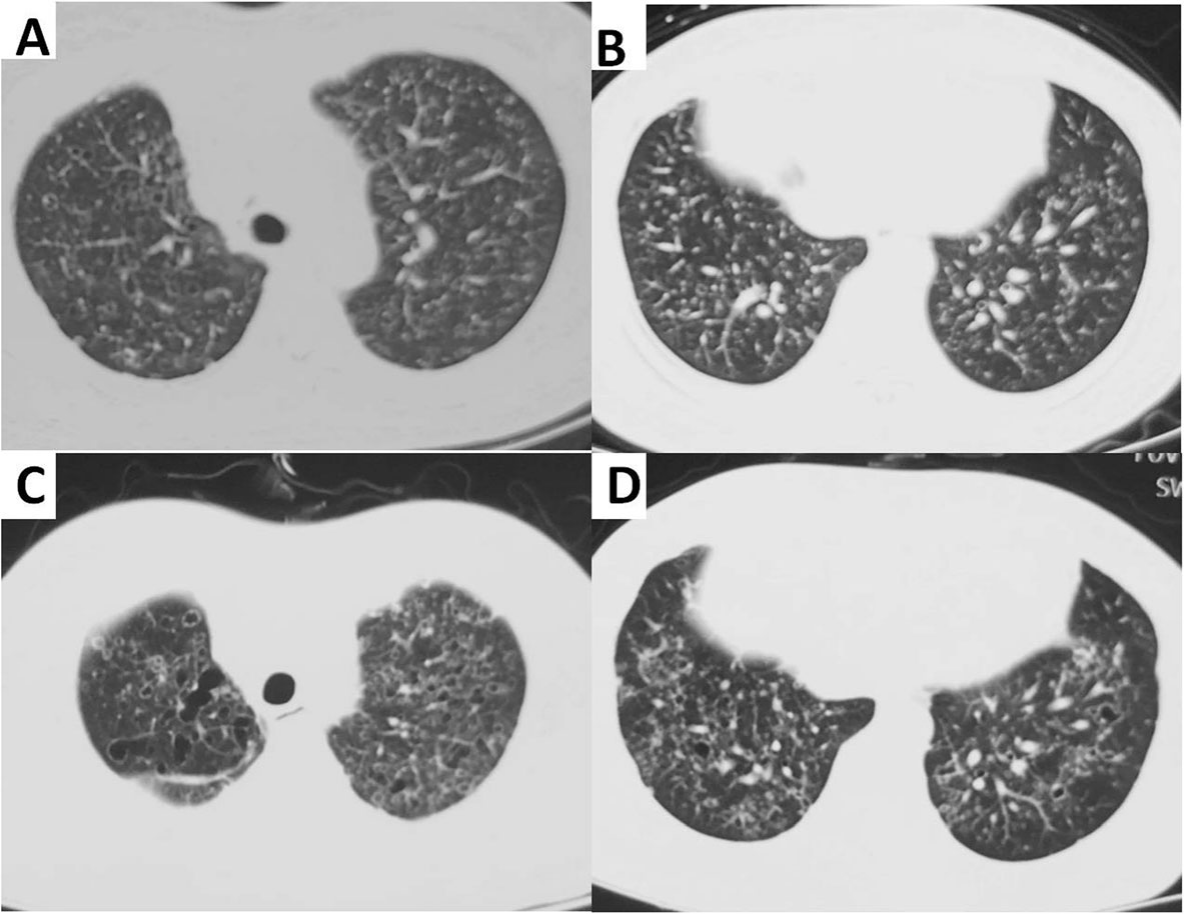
Computed tomography (CT) scan of the thorax. a, b CT of the thorax on February 2018 showed diffuse nodules and cysts distributing in bilateral lung. c, d Repeated Chest CT on August 2018 revealed enlarged, widespread, disseminated thin-walled cysts with different shape
